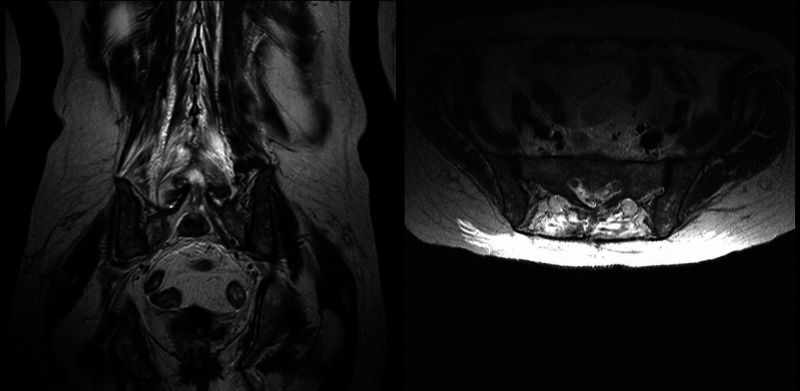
Sagittal and axial T1-weighted MRI obtained upon discharge showing no signs of intrapelvic abscesses on either side. MRI: magnetic resonance imaging
